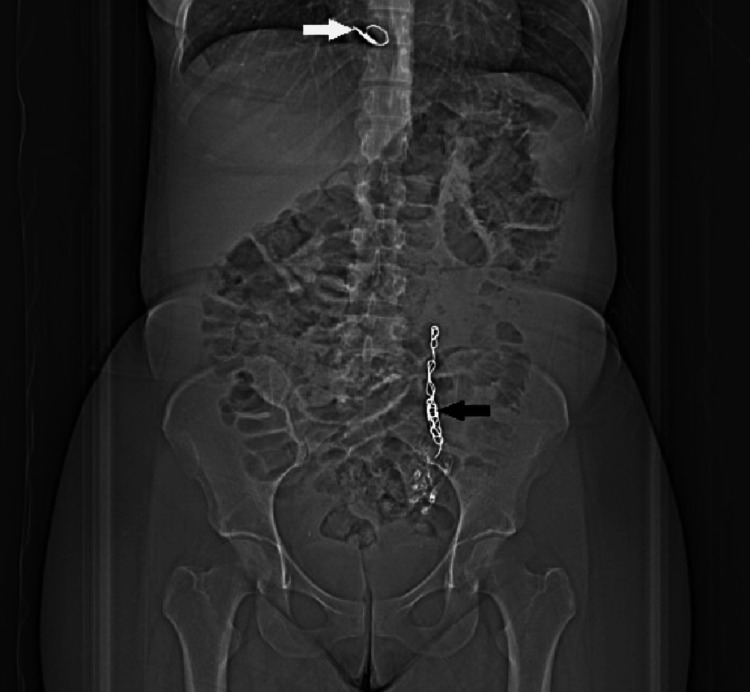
Plain radiography showing the endovascular coil positioned in the right ventricle after ovarian vein embolization The white arrow indicates the coil in the right ventricle and the black arrow indicates coils in the left ovarian vein.
